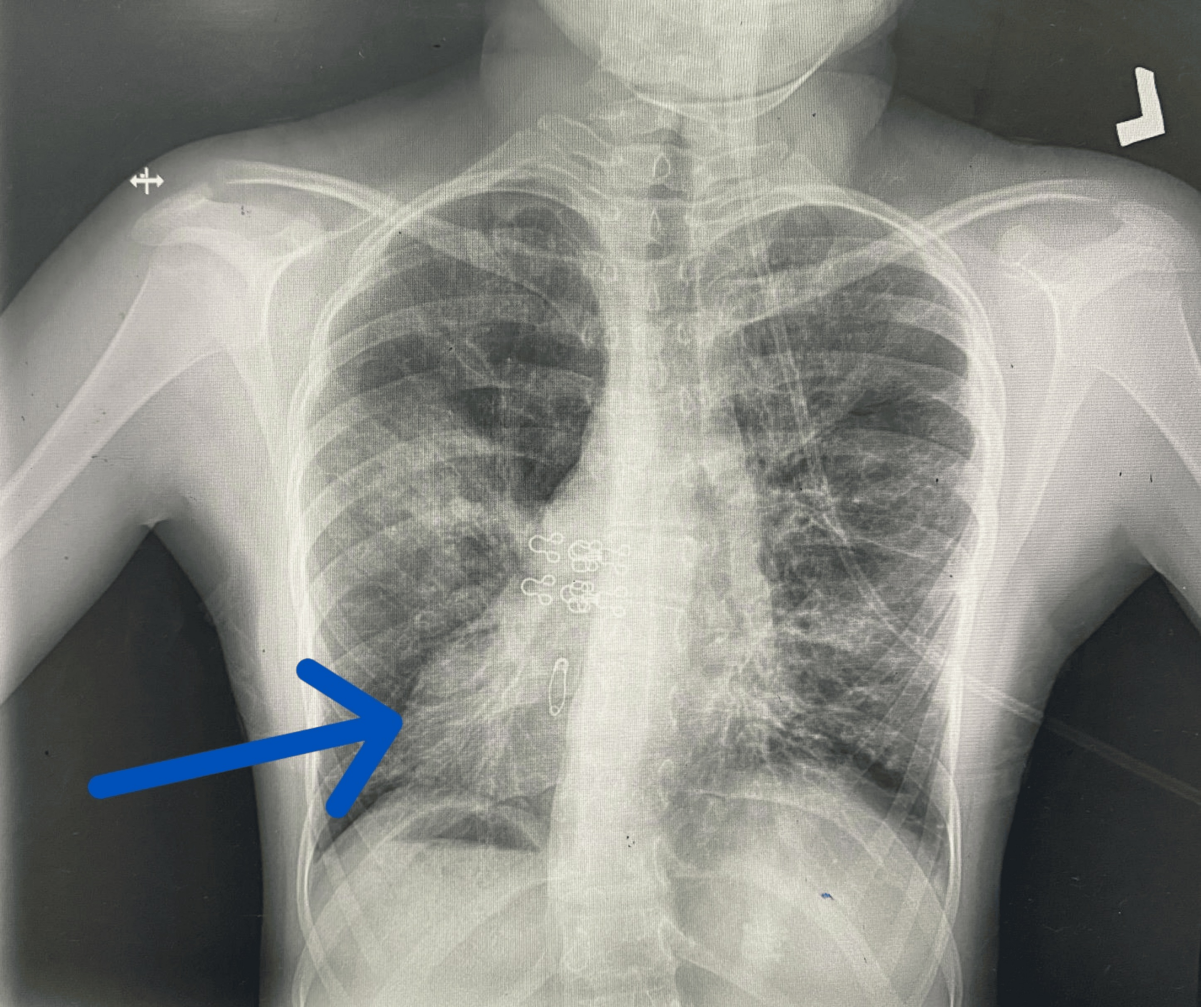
The patient's chest X-ray (posteroanterior view) demonstrates dextrocardia, with the cardiac apex oriented towards the right hemithorax, indicating right-sided heart positioning.
